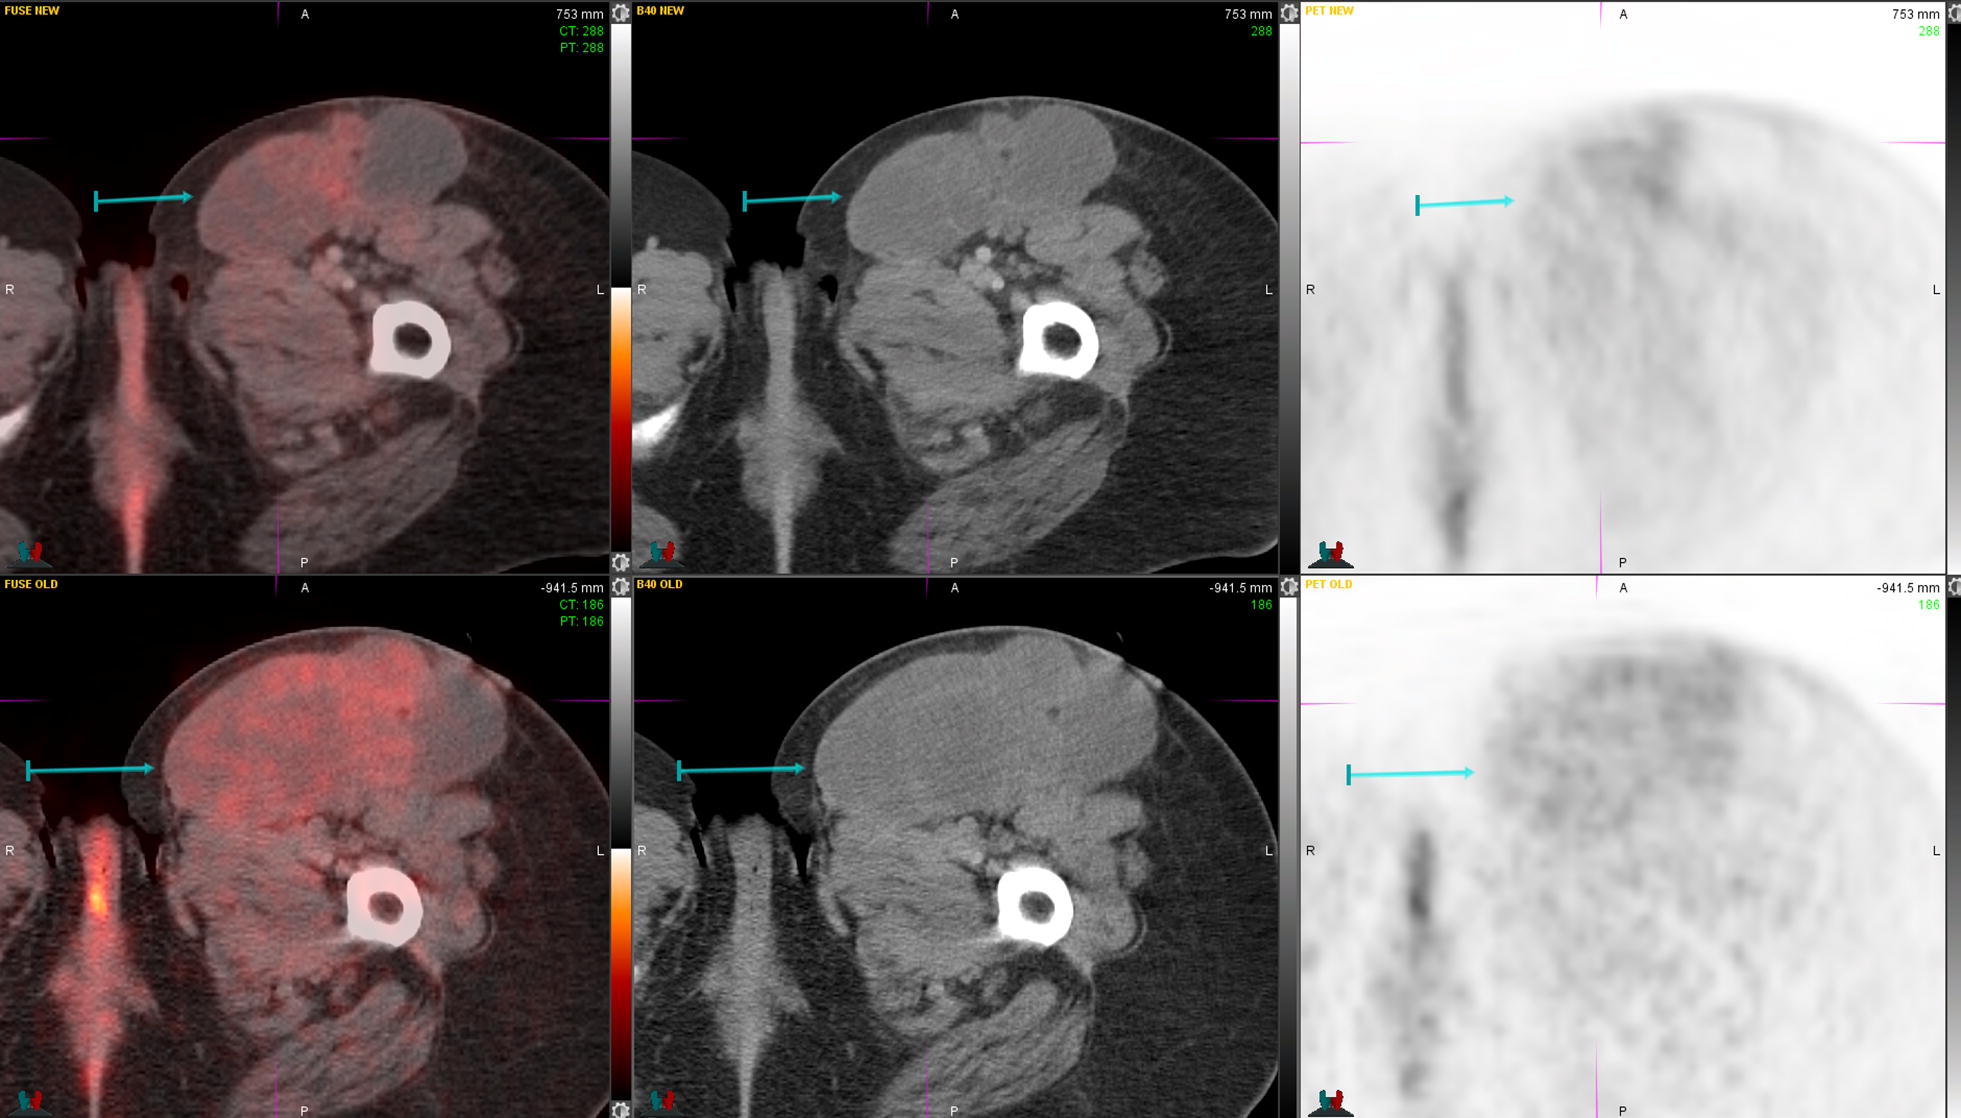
PET/CT before (lower images) and after (upper images) palliative radiation therapy to the left inguinal/thigh area
